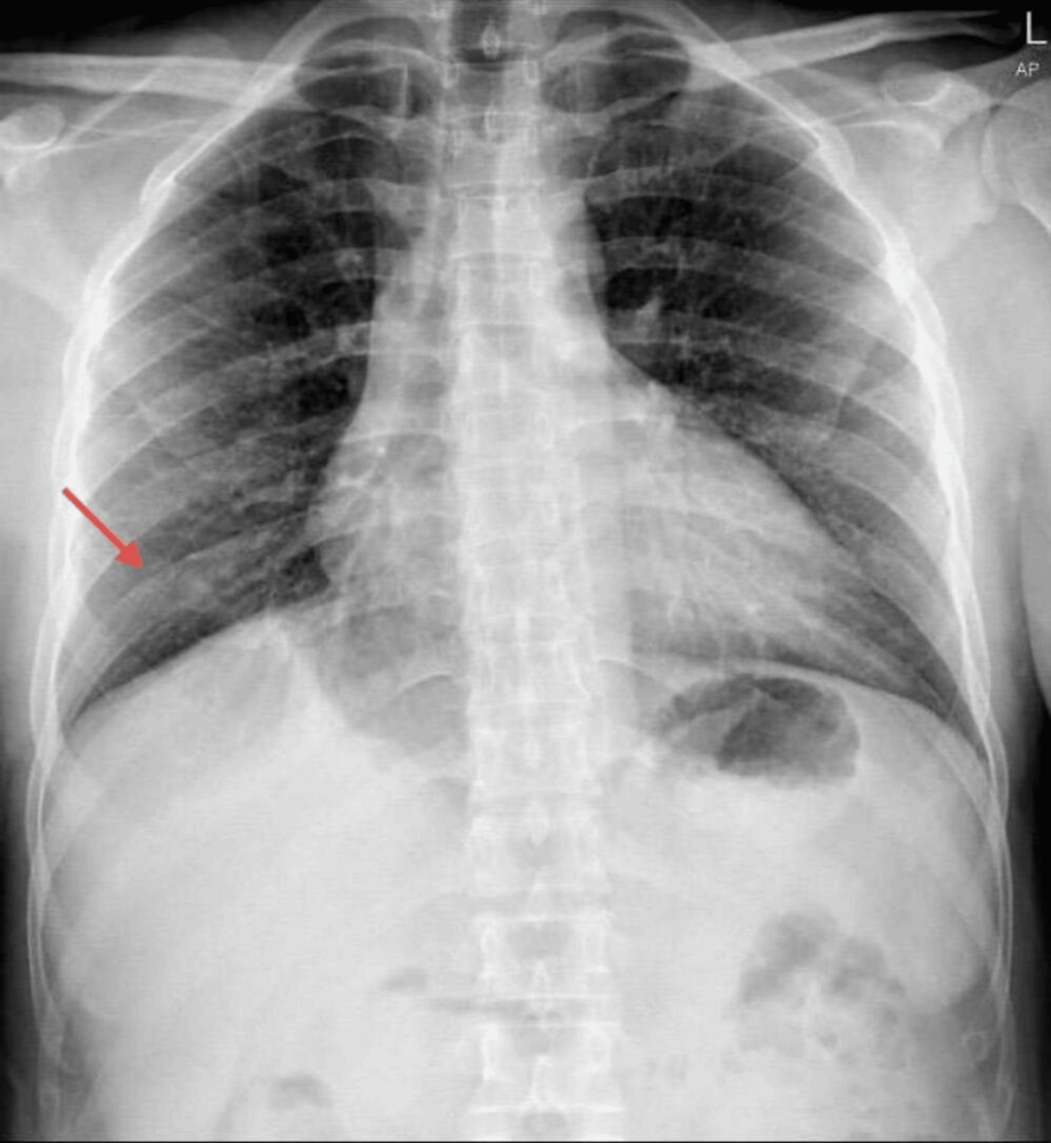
Chest X-ray - Patchy consolidation in the right lower lobe
